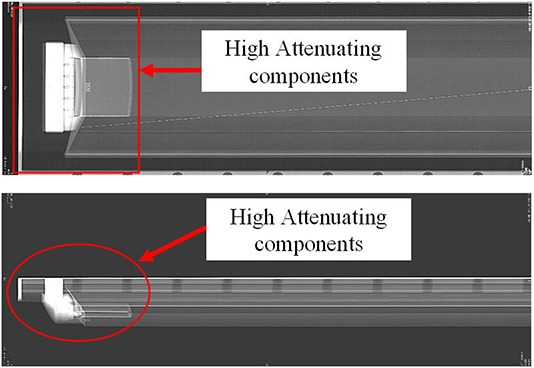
Scout views of a GE LightSpeed RT CT couch with MedTec fat top: (a) 0° view; and (b) 90° view.
